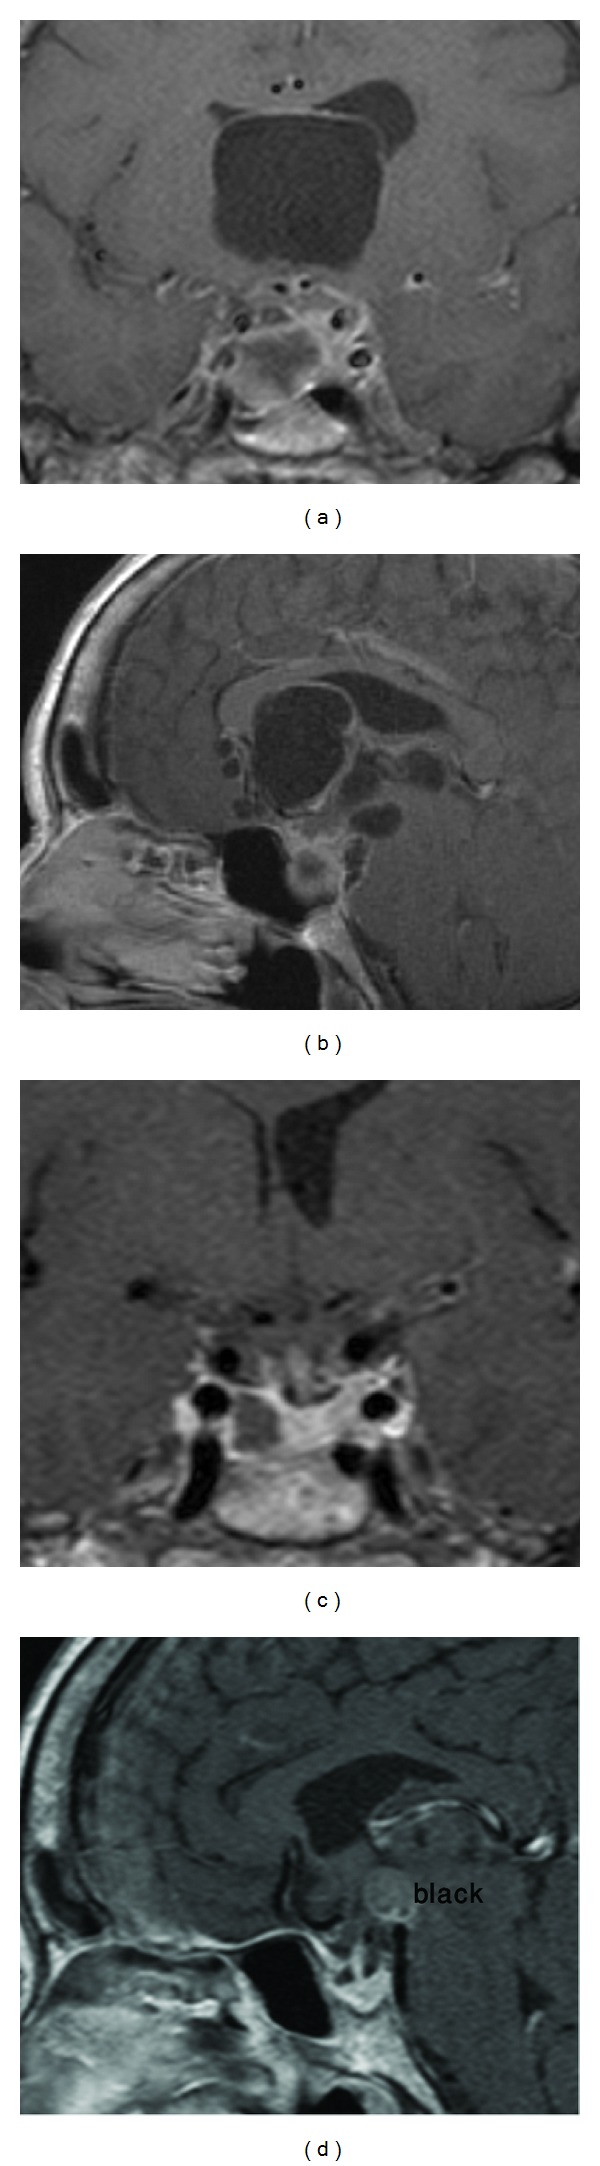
Preoperative. Coronal (a) and sagittal (b) T1-weighted MRI with gadolinium enhancement showing multiple cysts in the sellar, suprasellar regions, third ventricle, and basal cisterns. There is heterogenous contrast enhancement around the left anterior clinoidal process and in the sella turcica. Postoperative coronal (c) and sagittal (d) T1-weighted MRI with gadolinium enhancement showing partial empty sella, resection of the pituitary adenoma, anterior clinoidal meningioma, and the cysts.
